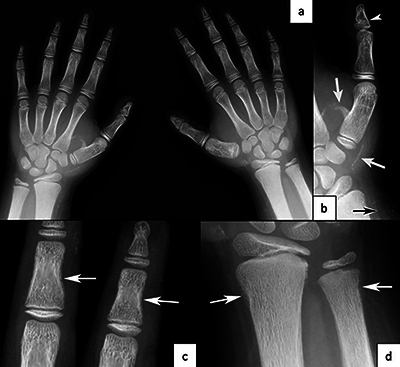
a) Hands radiography for bone age assessment. Slight brachymetacarpy of the 3rd, 4th and 5th metacarpals and brachyphalangy (P2R1/P2R5). b) Small distal phalanx of both thumbs due to premature fusion of growth plate (arrow head), pseudo-exostosis and linear ossification (arrows). c) and d) Resorption of subperiosteal bone (arrows).
